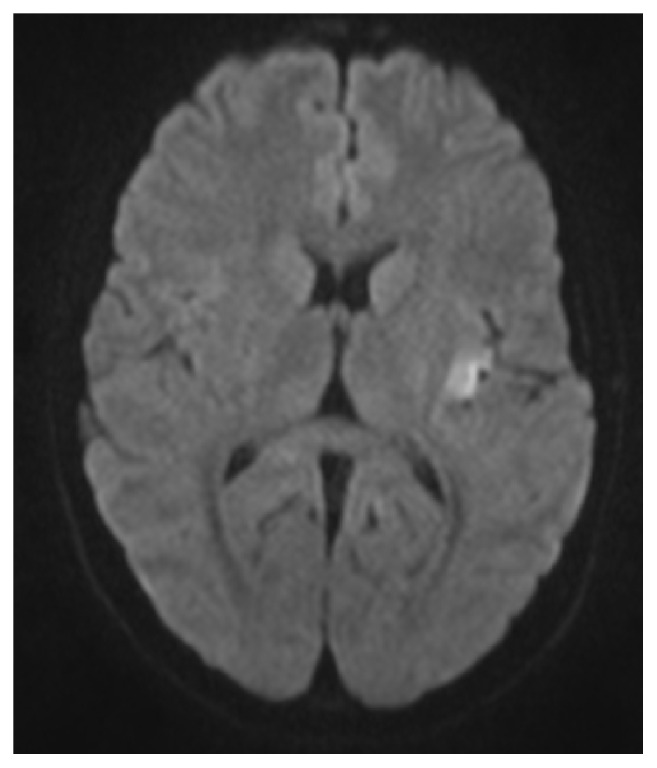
MRI brain: area of diffusion restriction in the left insula.
